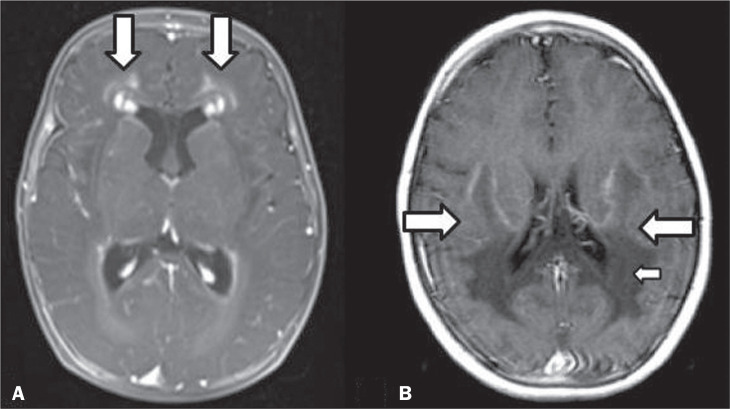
Contrast enhancement in INMDs. Contrast-enhanced MRI of the skull. A:
